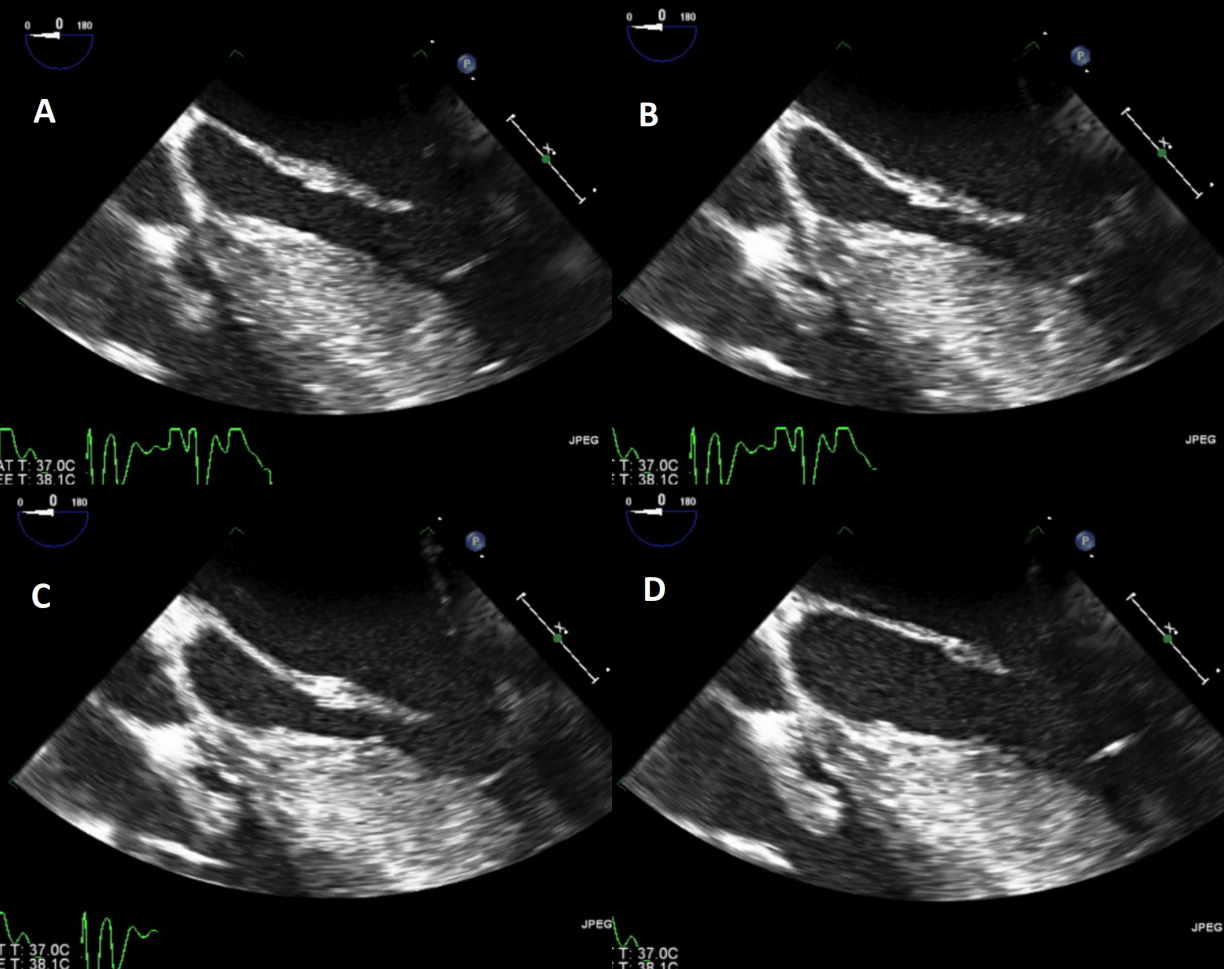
(A–D) Transesophageal echocardiogram at mid-esophagus showing
